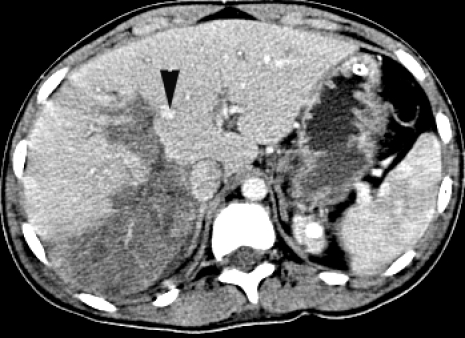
Abdominal CT. Degree Ⅳ liver laceration. Black arrowhead – middle hepatic vein.
